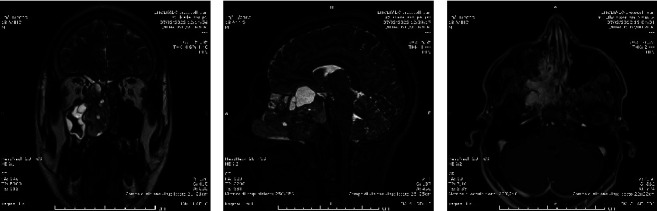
Preprocedural MRI scan: coronal (a) and sagittal (b) T2-weighted views showing large JNA of right nasopharyngeal cavity with expansion of the pterygopalatine fossa and extension into the infratemporal fossa with solid and cystic component. (c) Gd-enhanced T1 gradient-echo 3D axial image showed early and strong diffuse enhancement of the lesion.
